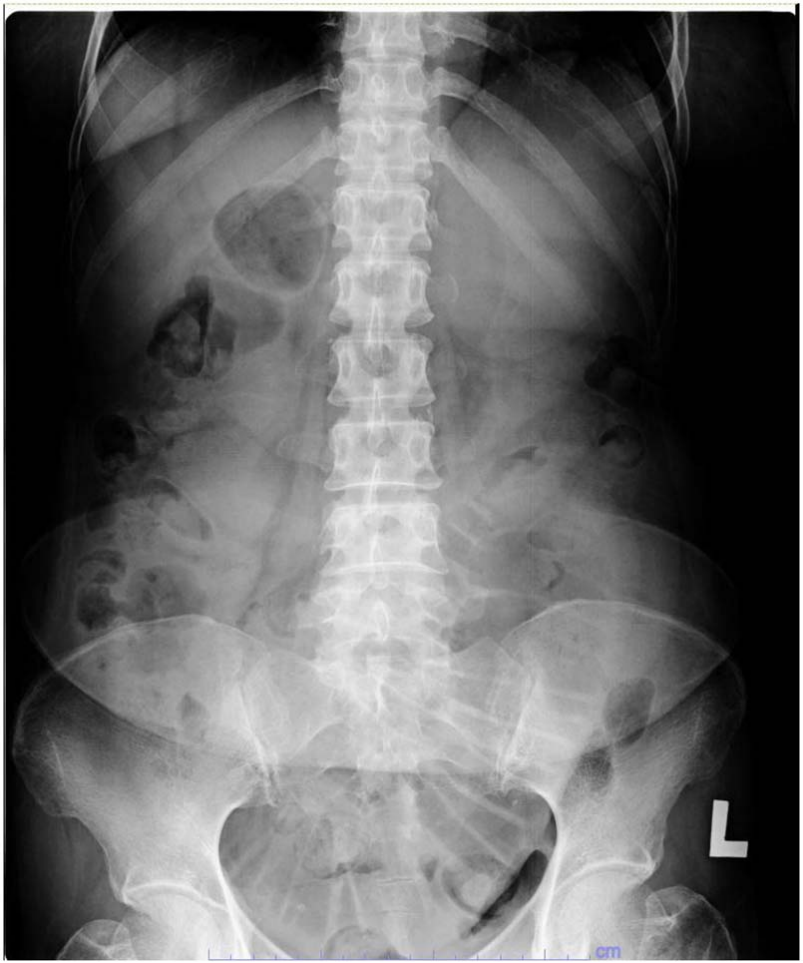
Abdominal x-ray showing a dilated small bowel with valvulae conniventes.
